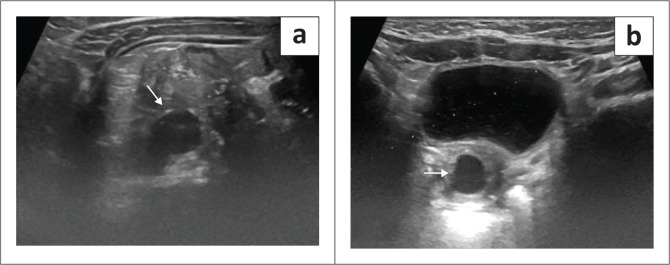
(a) Transverse ultrasound image revealed an ectopic dysplastic right kidney (*white arrow*) in the umbilical region. (b) Transverse ultrasound image showed a focal cystic dilatation of the right tubulocystic ureter posterior to the bladder (white arrow). The insertion of ureter was not well visualised.
